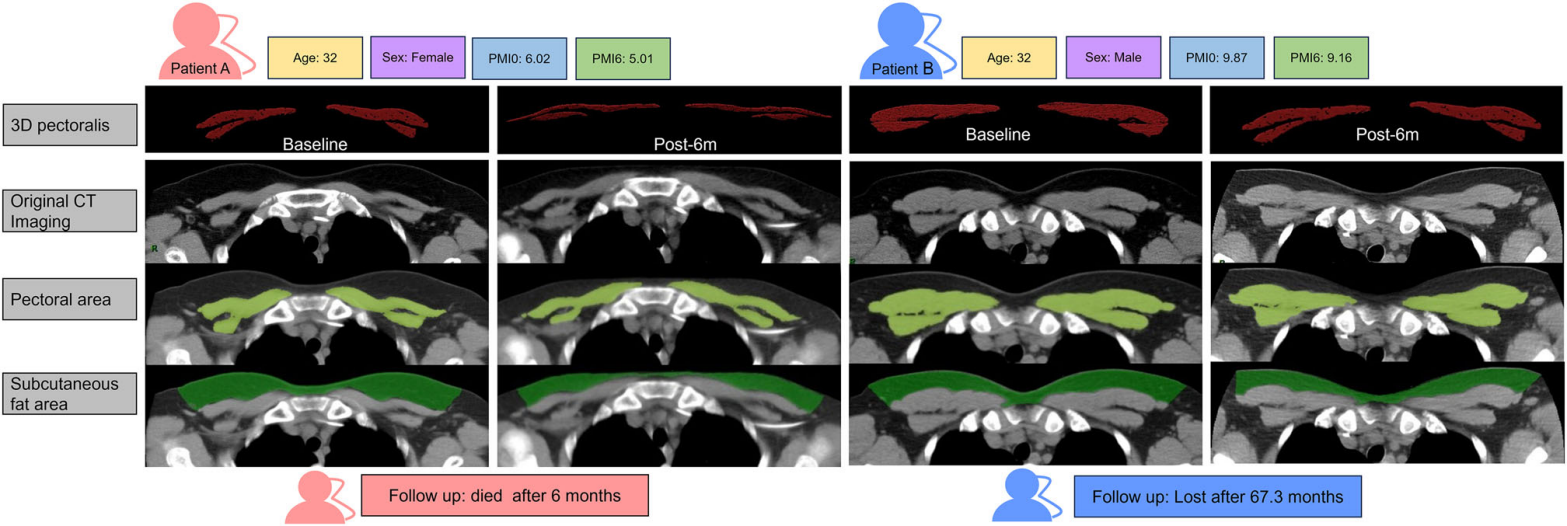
On axial unenhanced chest CT images, changes in the pectoral muscle area (CT thresholds ranging from −29 to 150 HU, green) and fat mass area (CT thresholds ranging from −190 to −30 HU, bottle green) were observed in sections above the aortic arch in SAA patients pre‐ and post‐ 6 months after HSCT. Patient A: A 32‐year‐old female with sarcopenia (PMI = 6.02 cm^2^/m^2^) at baseline, and a decreased PMI of 5.01 cm^2^/m^2^ after 6 months of HSCT; the patient died after 6 months of follow‐up. Patient B: A 32‐year‐old male with nonsarcopenia (PMI = 9.87 cm^2^/m^2^) at baseline and remaining nonsarcopenia with slight muscle loss post‐6 months (PMI = 9.16 cm^2^/m^2^) was included; the patient was lost to follow‐up after 67.3 months.
